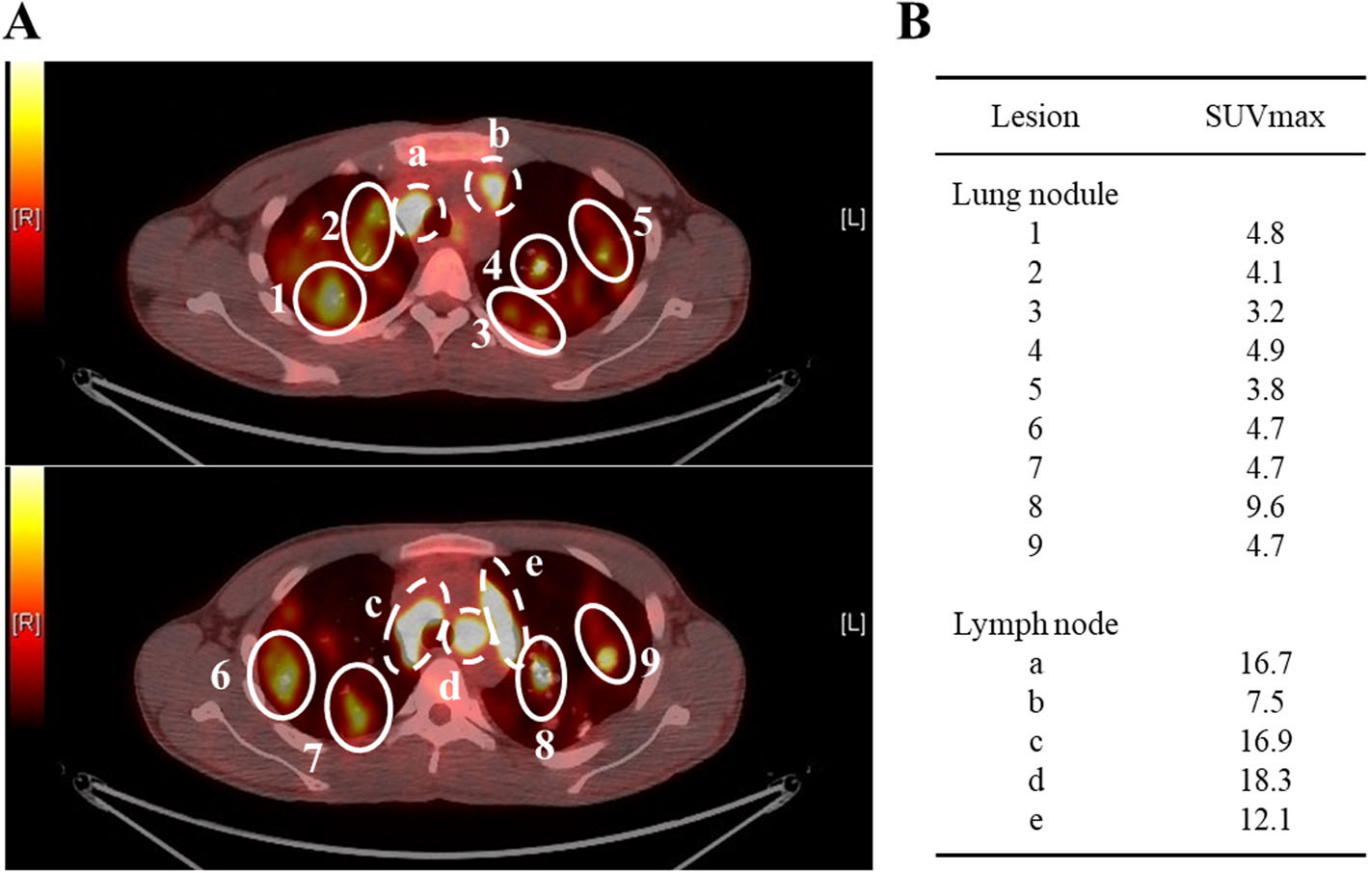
The axial view of FDG-PET/CT scan on diagnosis. (A) PET/CT scan shows intense FDG uptake in bilateral lung masses and the mediastinal and hilar lymph nodes. Each lung nodule is surrounded by a solid-line circle (1–9). Each mediastinal lymph node is surrounded by a dotted line circle (a-e). (B) The maximum standardized uptake values (SUVmax) of the lesions are shown here
